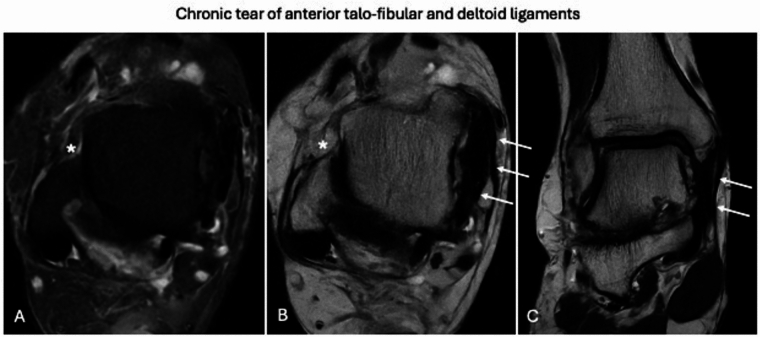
Chronic tear of anterior talo-fibular and deltoid ligaments. Ankle MRI performed four months after trauma shows anterior talo-fibular ligament atrophy, which is unidentifiable on axial fat-suppressed proton density weighted (A) and T2-weighted (B) images. The hypointense fibroscar thickening of deltoid ligament can be recognized on axial T2-weighted (B) and coronal T2-weighted (C) images. Neither bone edema nor peri-capsular edema can be seen on axial fat-suppressed proton density weighted (A) image
